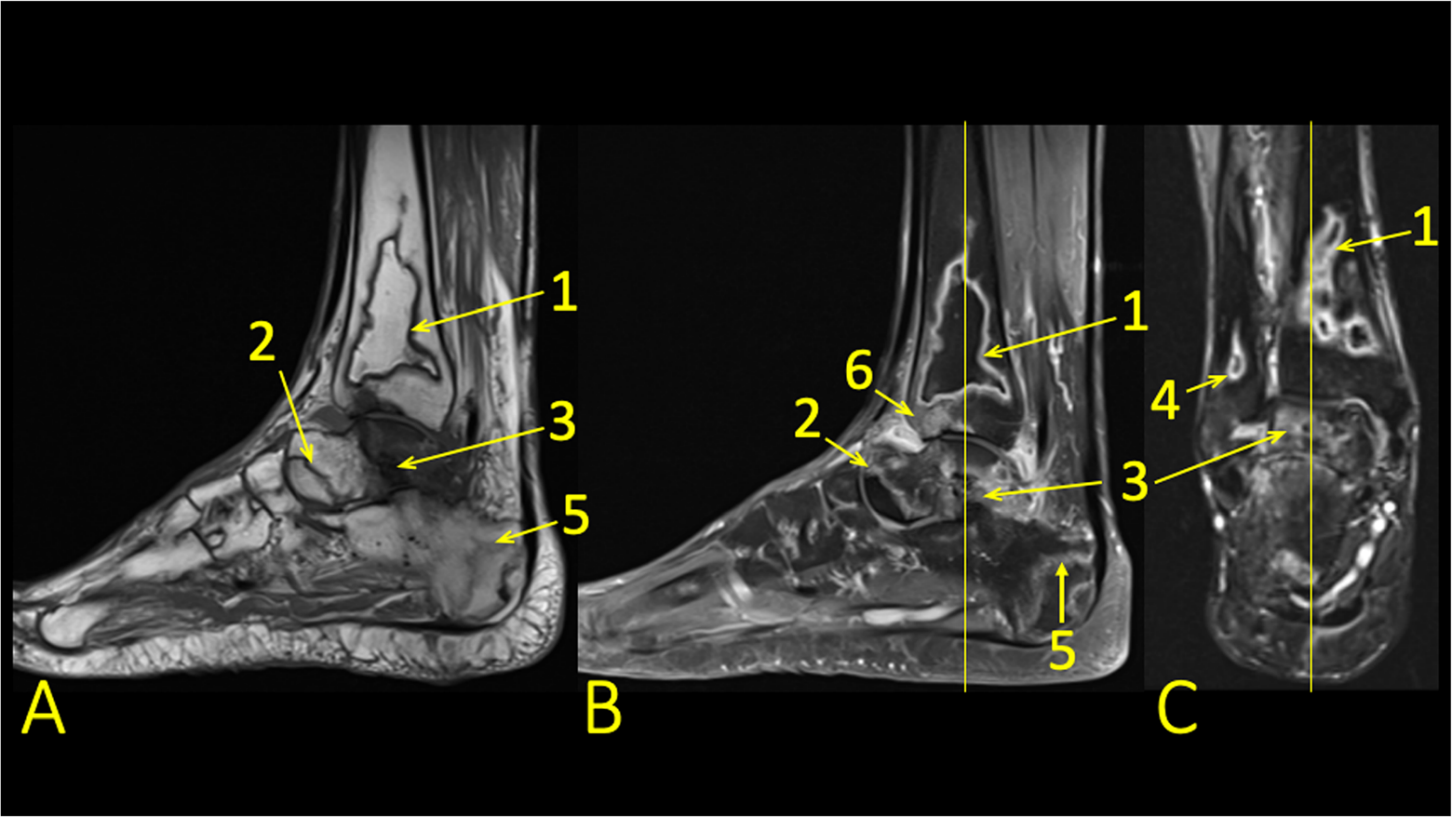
A 45-year-old male, 2 years after kidney transplantation, presented with 6-week history of ankle pain and a suspected talar stress fracture. MRI showed bone infarcts in the distal tibia (1), fibula (4), talus (2), and calcaneus (5) and a talar fracture (3). BME in the subchondral part of the distal tibia (6)
